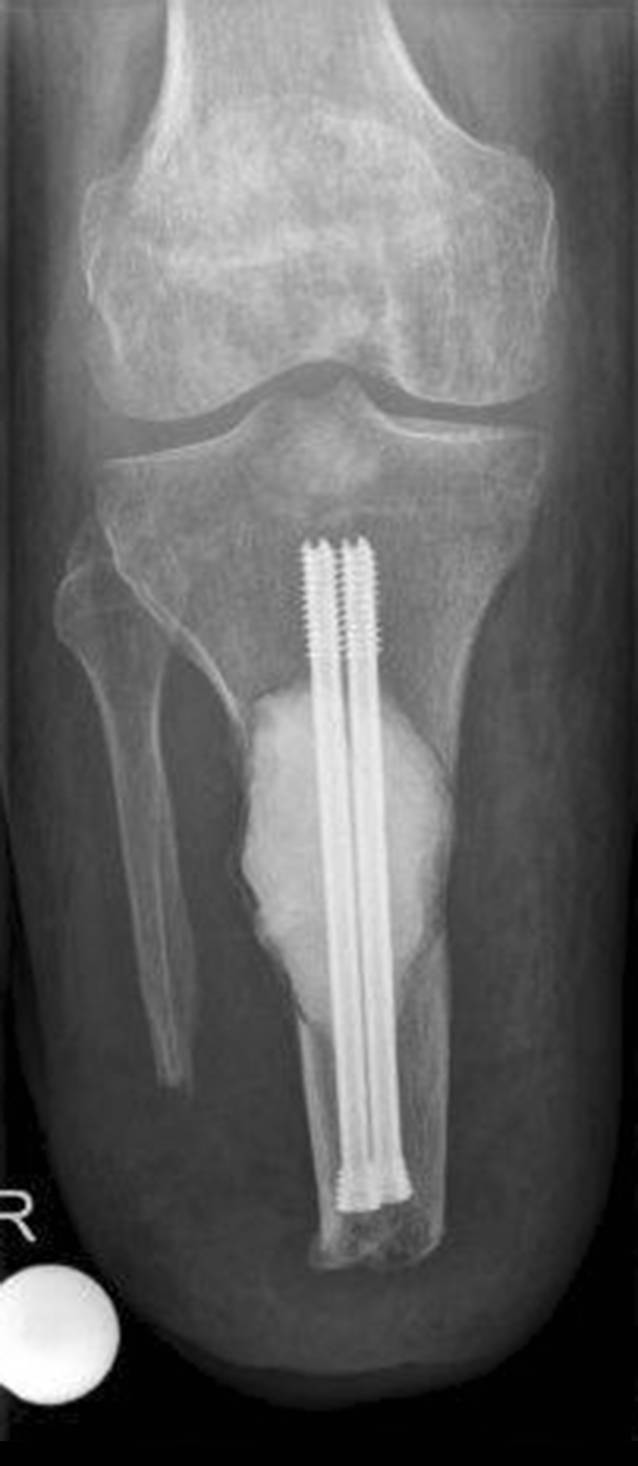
Eine planmäßige Wiedervorstellung zur klinisch-radiologischen Verlaufskontrolle erfolgte 3 Monate postoperativ. Nativradiologisch und MR-graphisch zeigte sich ein konstanter Befund ohne Hinweis auf ein lokales Tumorrezidiv (Abb. 6). Eine pulmonale Manifestation wurde aufgrund der aggressiven Biologie des hier beschriebenen TSGCT als Einzelfallentscheidung CT-graphisch ausgeschlossen. Der Patient berichtete im Rahmen dieser Konsultation bereits über eine vollständige Remobilisation mit unlimitierter Gehstrecke. Er konnte seinen Alltag wieder ohne Einschränkungen bewältigen. Die Narbe war reizlos verheilt und der Stumpf regelrecht konfiguriert (Abb. 7). Der Patient demonstrierte mit angelegter Exoprothese (Abb. 8) ein flüssiges und weitestgehend physiologisches Gangbild (siehe Video online zu diesem Beitrag).
